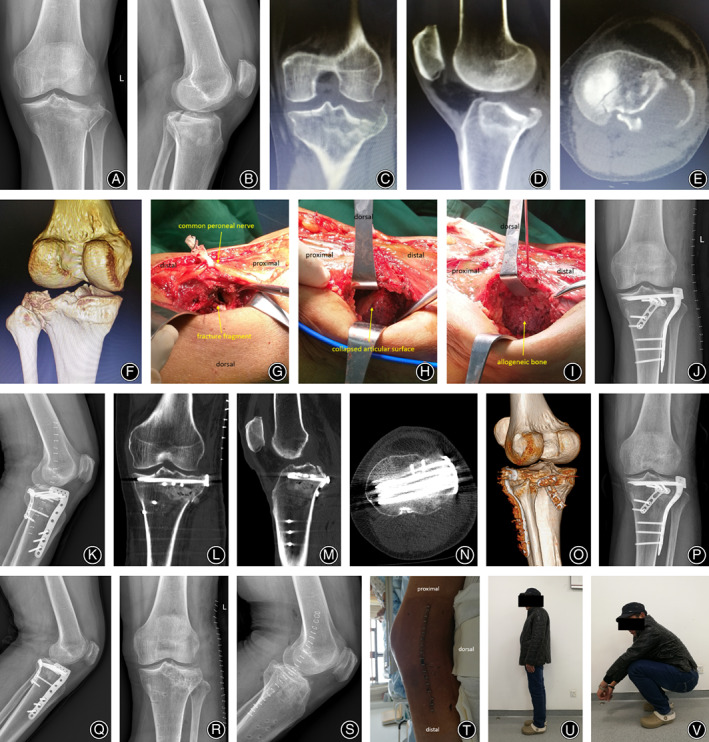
Typical case 1. A 58‐year‐old man was injured from a beating. (A–F) Preoperative X‐ray and CT images show a posterolateral tibial plateau fracture combined with a lateral tibial plateau fracture. (G–I) During the operation, the articular surfaces of the posterolateral tibial plateau and the lateral tibial plateau are obviously collapsed. After anatomical reduction of the articular surface, allograft bone is implanted in the bone defect area. (J–O) Postoperative X‐ray and CT images show an anatomical reduction of the tibial plateau fractures. (P, Q) Ten weeks after the operation, anteroposterior and lateral radiographs of the knee show that the fracture is completely healed. (R, S) One year after the operation, the internal fixation was removed. (T) The appearance of the patient's surgical incision. (U, V) The function of the patient's knee joint at the last follow‐up. (In this case, the posterolateral tibial plateau fractures were mainly the collapse of the posterolateral articular surface).
